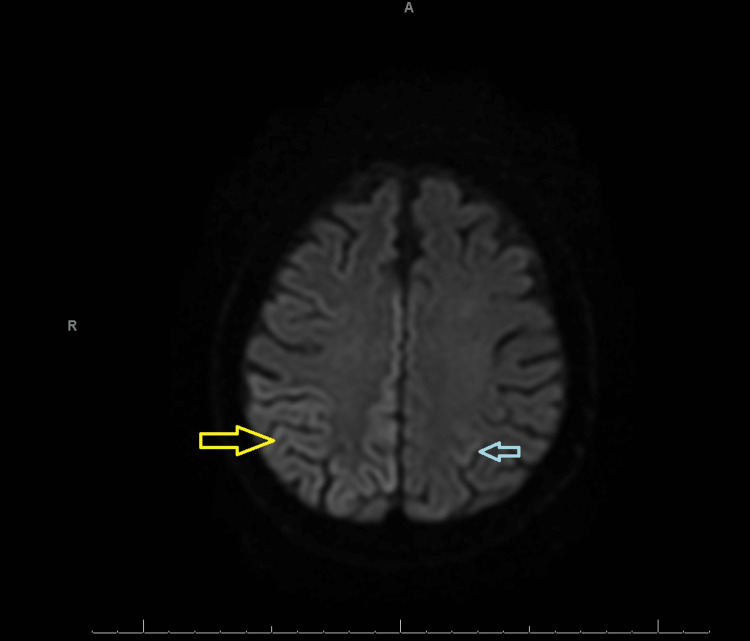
MRI of the brain without contrast (DWI) displaying areas of curvilinear hyperintense signal in the right parietal, frontal, and temporo-occipital cortex (yellow arrow*) and to a lesser extent in the left parietal cortex (blue arrow) *Yellow arrow displays the area of restricted diffusion in the right occipital cortex. DWI: Diffusion-weighted imaging.
